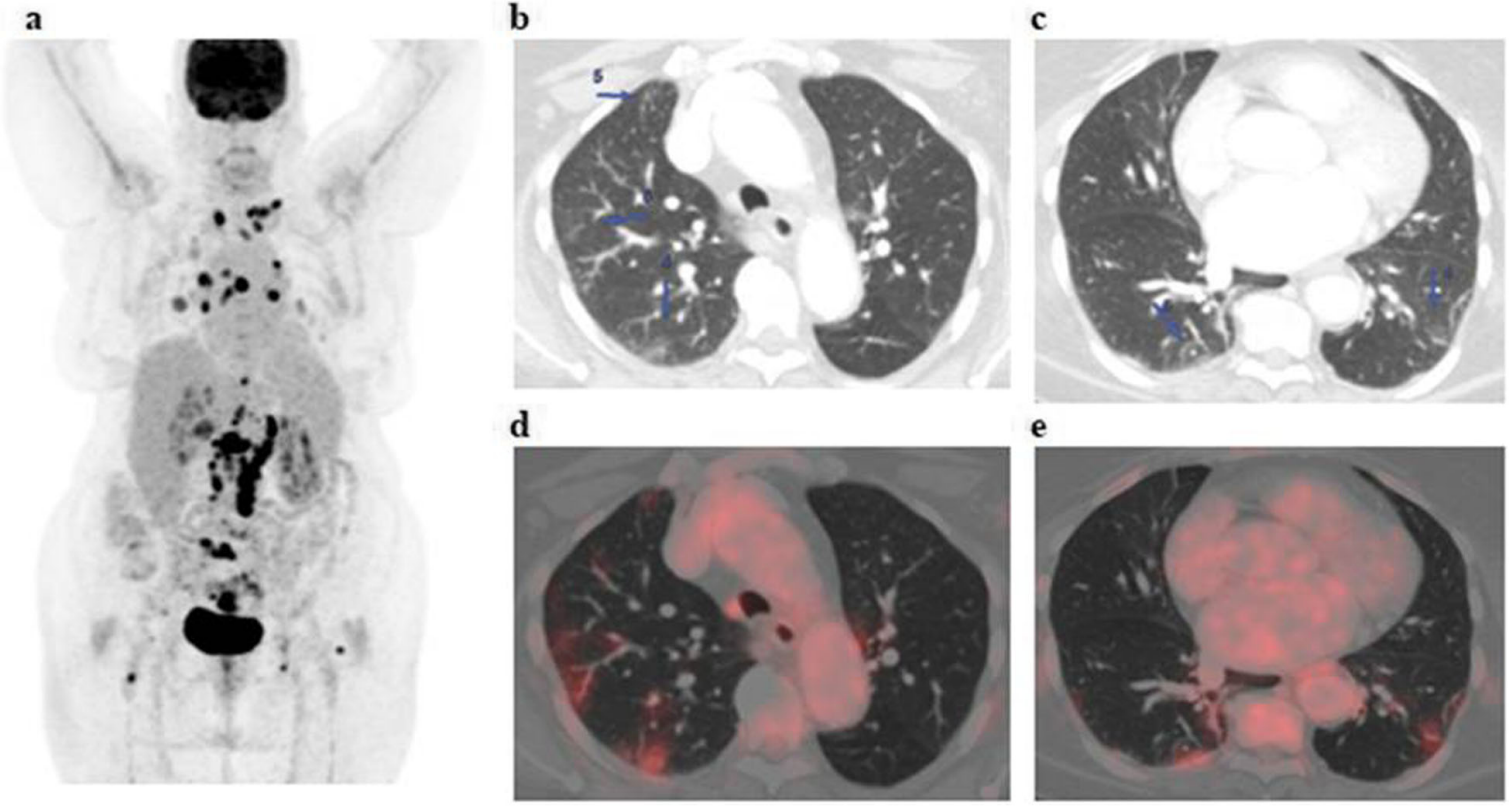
A 74-year-old female patient, presented with low back pain and previously done lumbosacral spine MRI which revealed malignant looking osseous lesions and referred for further assessment by PET/CT examination. The whole-body MIP image (a) shows multiple FDG avid nodal and osseous lesions with bilateral pulmonary areas of mild active FDG uptake. Axial lung window CT images (b, c) revealed small-sized bilateral subpleural ground-glass opacities that show mild FDG avidity on corresponding fused PET/CT images (d, e) achieving up to 4.3 SUVmax
